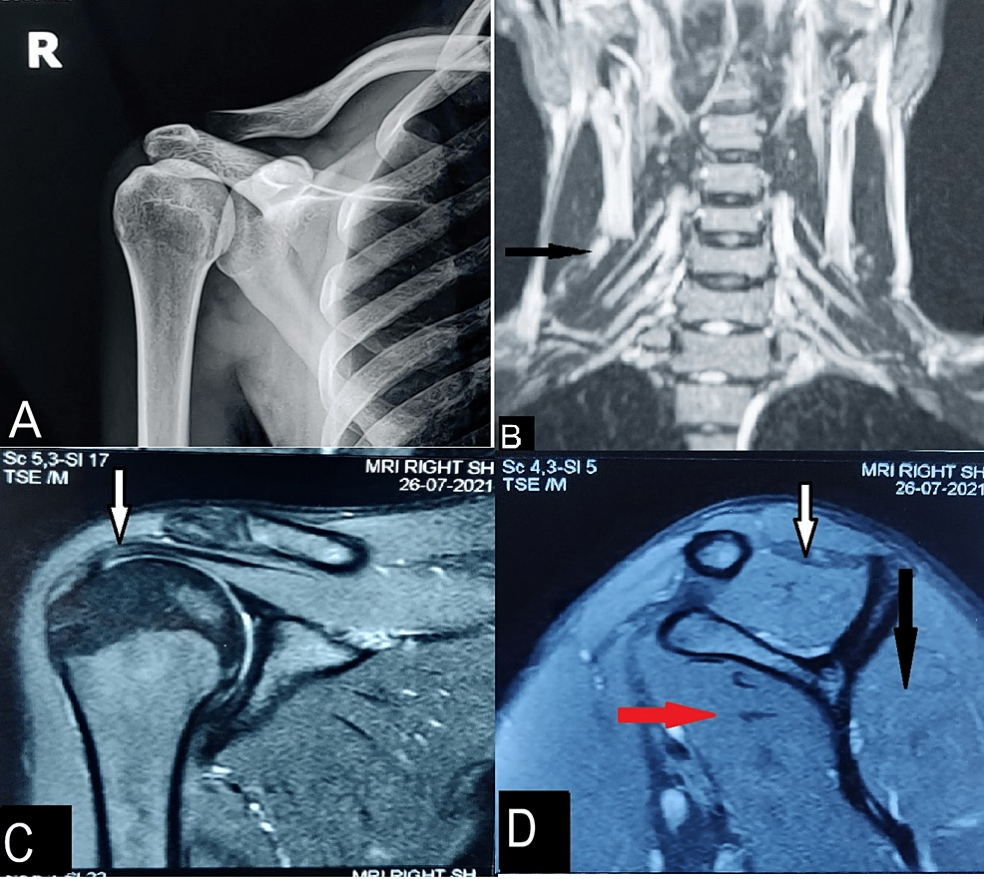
Radiological images at five weeks post-vaccination Normal X-ray anteroposterior view of the affected shoulder (A). Neurogram of upper brachial plexus showing intact nerves (B). T1 coronal MRI image of affected shoulder showing intact tendon (C). T1 sagittal MRI image showing normal supraspinatus, infraspinatus, and subscapularis muscle bulk represented with white, black, and red arrows respectively (D).
